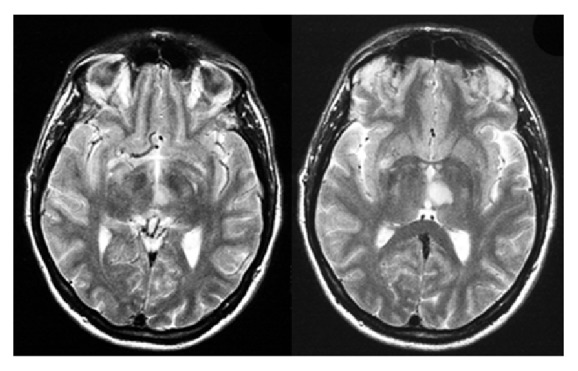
Transversal brain MRI (FLAIR) images of the patient AB showing the acute ischemic lesion in the medial dorsal nucleus of the left thalamus.
